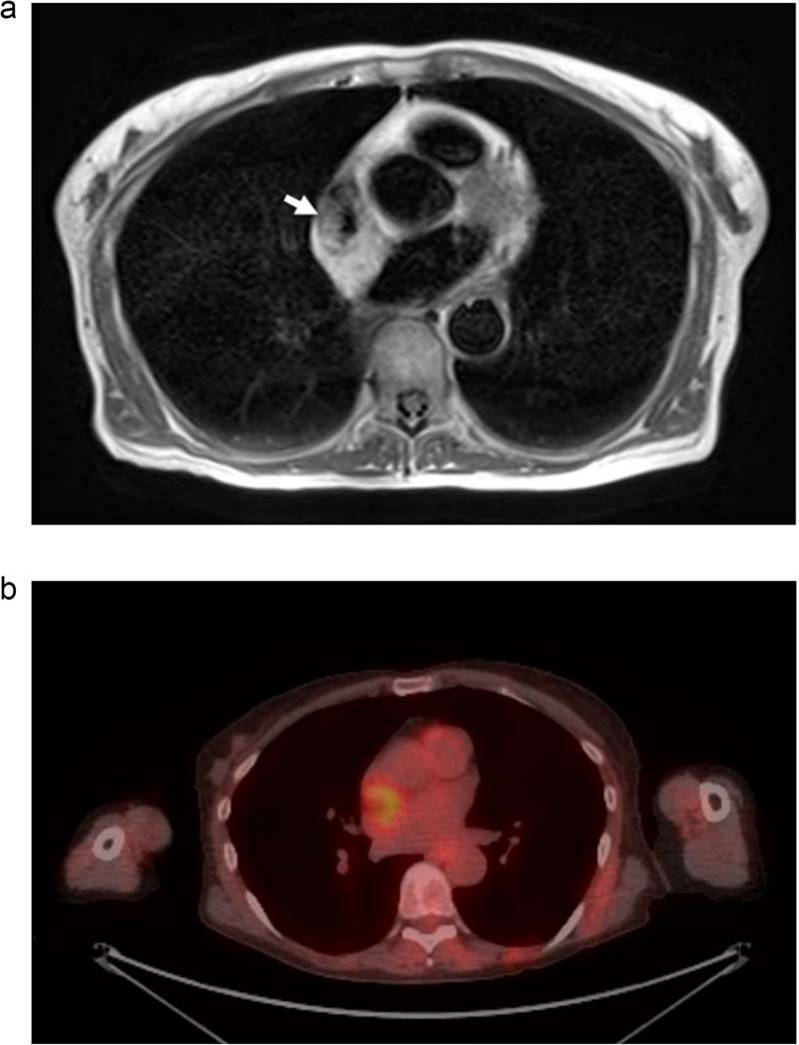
(a) MRI chest. Mass represented by white arrow. (b) PET/CT. Uptake is demonstrated in the mass associated with the SVC and right atrium.
